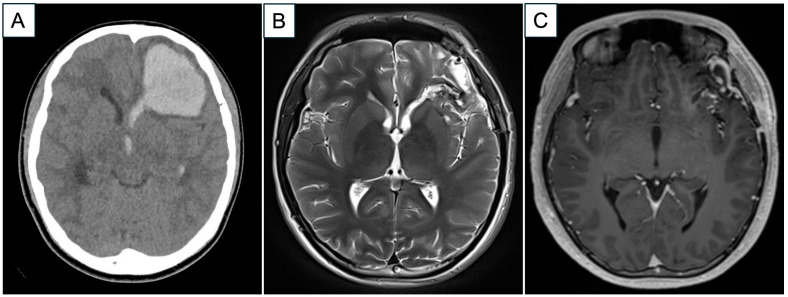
(A) Representative CT image in axial direction depicting a large, acute left frontal hematoma with intra-ventricular extension. (B) Representative MRI axial image in T2-weighted sequence post-ICH evacuation. There is improvement in the local mass effect. (C) Representative post-contrast T1-weighted MRI axial image that shows a likely vascular malformation in the left antero-temporal region. (Abbreviations: CT = computed tomography, ICH = intracerebral hematoma, MRI = magnetic resonance imaging).
